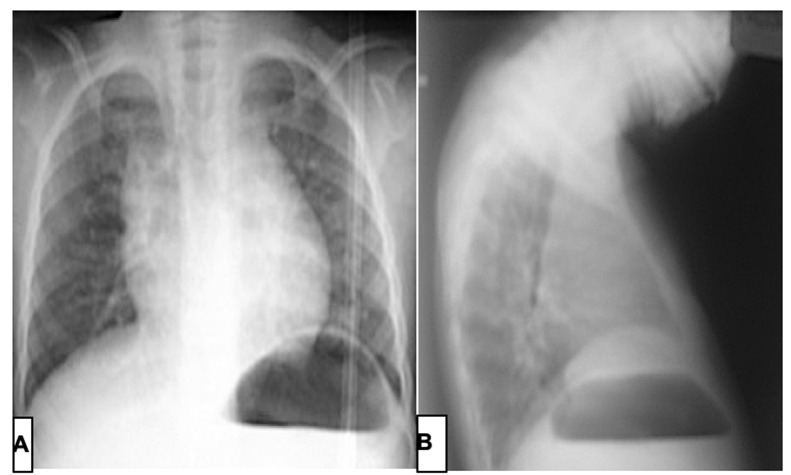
A) radiographies du thorax; B) face; profil
